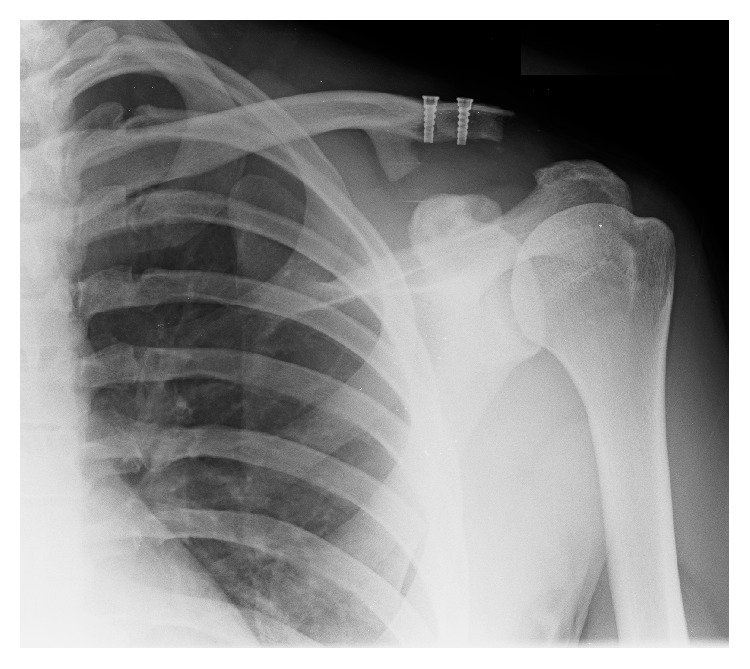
Anteroposterior left ACJ radiograph at three months after LARS July 2016 implantation demonstrating relapse of ACJ dislocation and heterotopic ossification medial to the medial clavicular screw.
